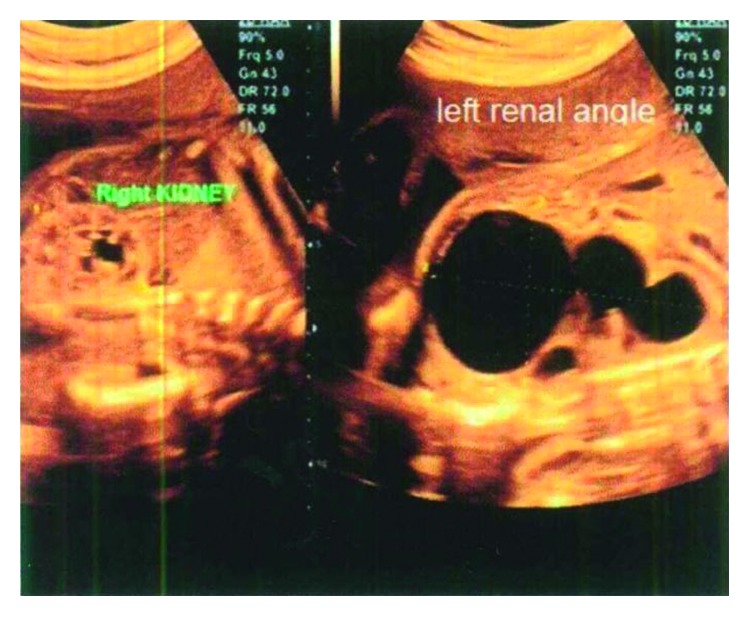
Antenatal ultrasound showed left-sided pelvic-ureteral junction stenosis.
